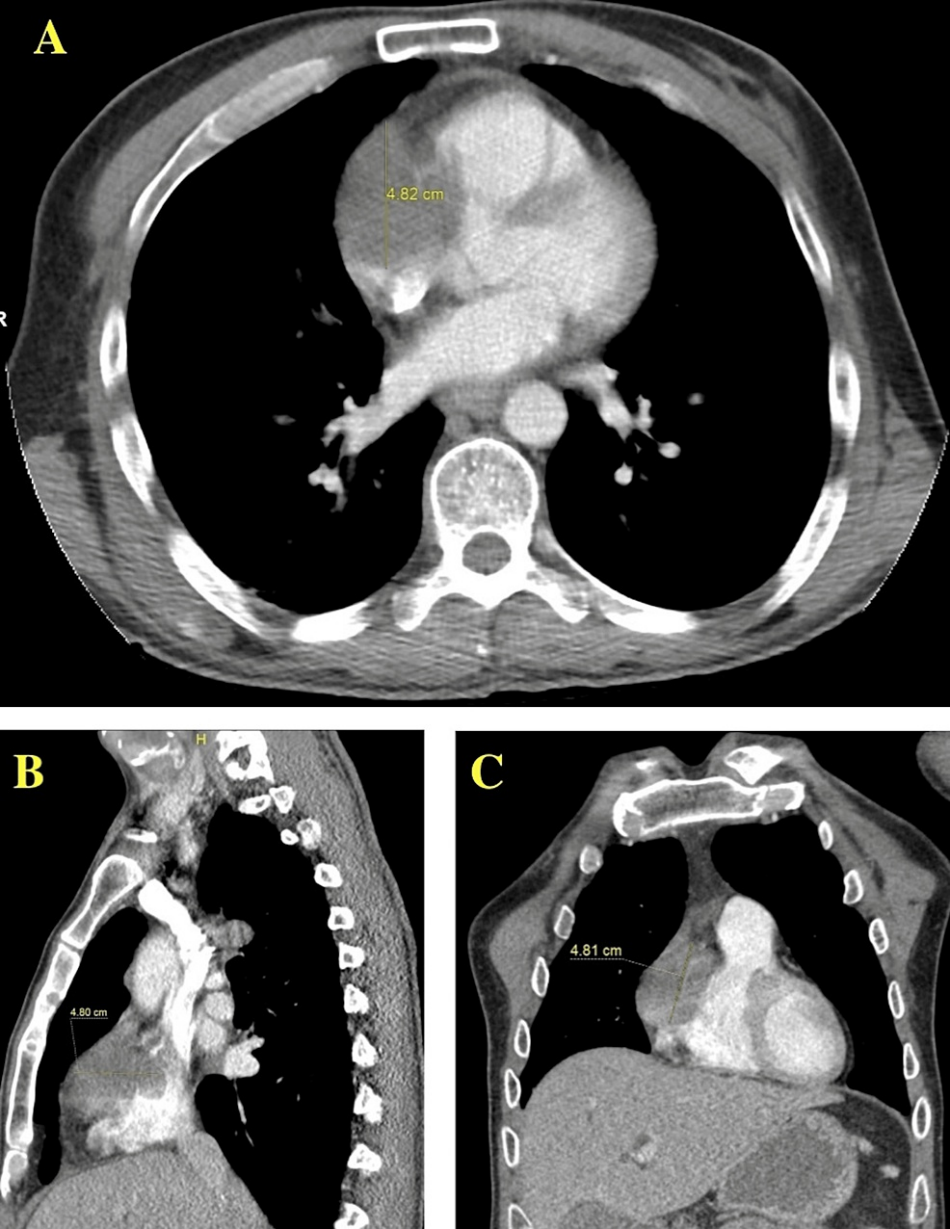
Chest CT with panels A, B, and C showing a 4.8 cm mediastinal mass. CT, computed tomography
